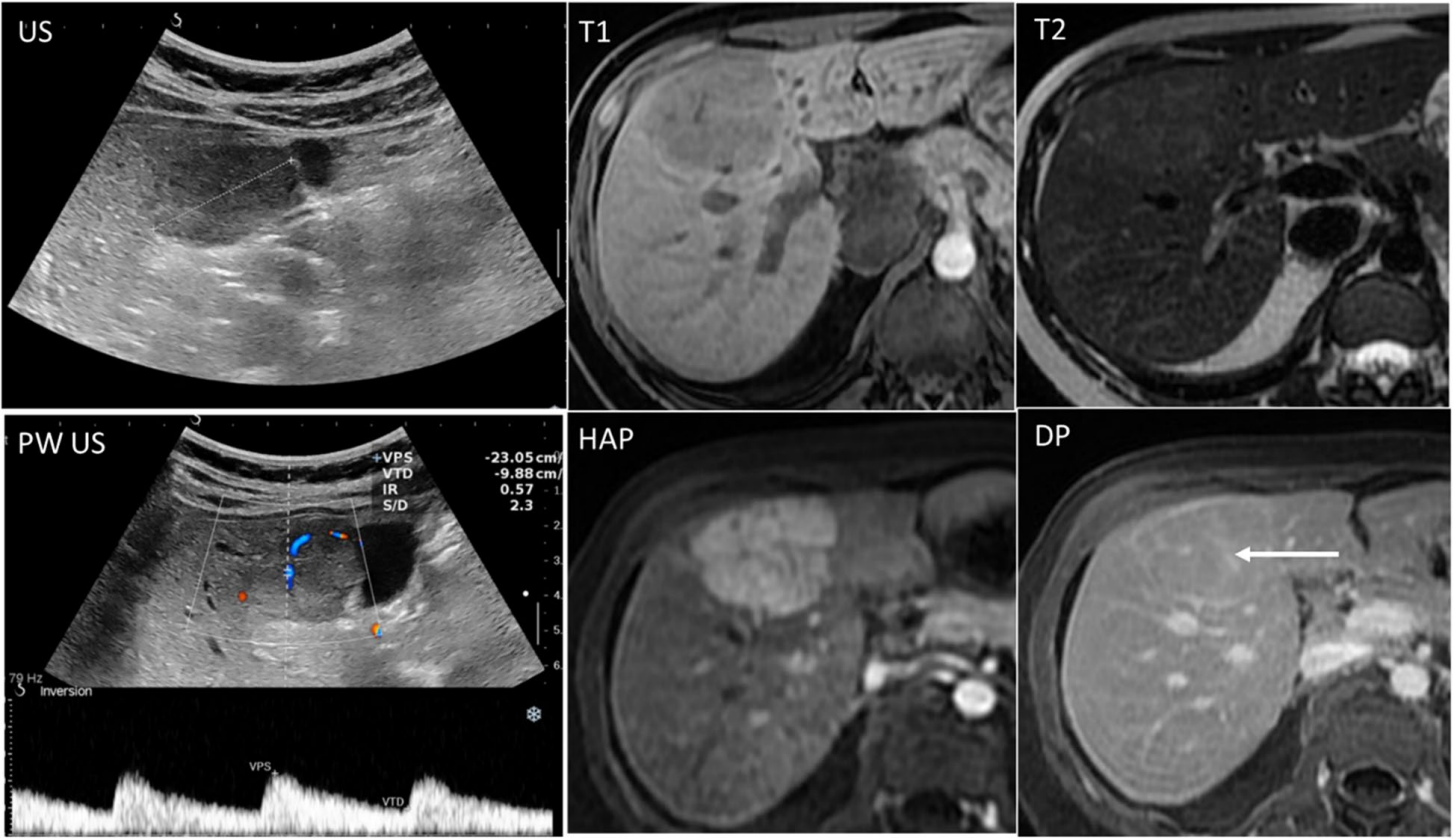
Focal liver lesion management in a 27-year-old pregnant woman. During routine US, a liver mass is seen (first picture, upper row), showing vascularization and arterial flow on Doppler US (first picture, lower row). MR study was requested and demonstrates a T1 hypointense lesion, slightly hyperintense on T2. This lesion was not deemed malignant therefore contrast-enhanced MRI was withheld until childbirth and showed avid enhancement on hepatic arterial phase (HAP) with homogenization on delayed phase (DP) and a central scar (arrow), typical of focal nodular hyperplasia
